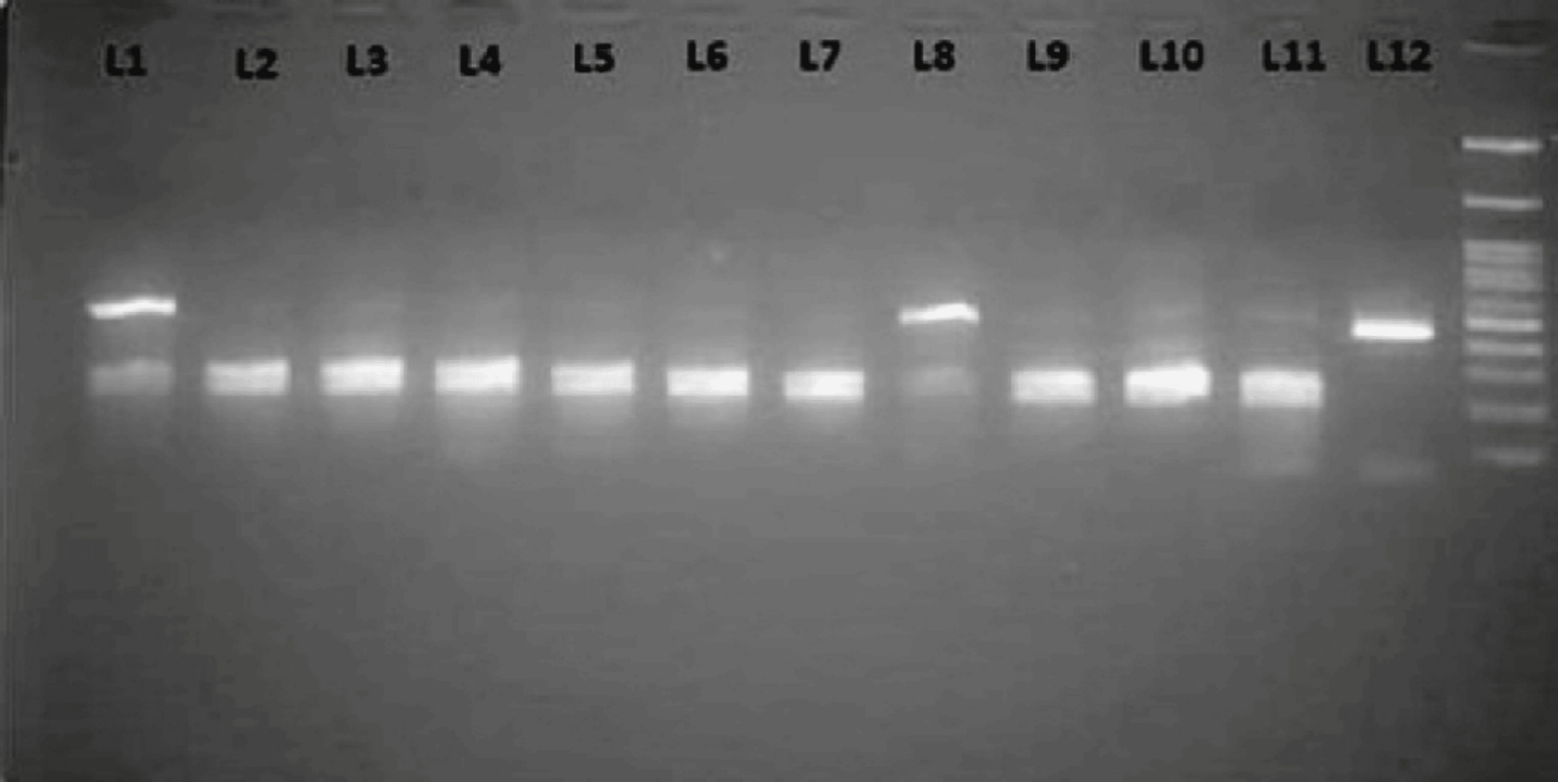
PCR product restriction digestion assay for the HIF1A gene's 1772C>T polymorphism using the HphI enzyme, visualized on a 2% agarose gel. A typical depiction of PCR product restriction digestion for the hypoxia-inducible factor 1 subunit alpha gene's 1772C>T polymorphism, employing the HphI enzyme, was distinguished on a 2% agarose gel. The CT genotype showed three bands (216, 251, 467 bp) in Lanes 1, 8, 10, and 11. The CC genotype showed two bands (216, 251 bp) in Lanes 2-7 and 9. The TT genotype appeared as a single 467 bp band in Lane 12. A 100 bp ladder was included in the last lane for size comparison.
